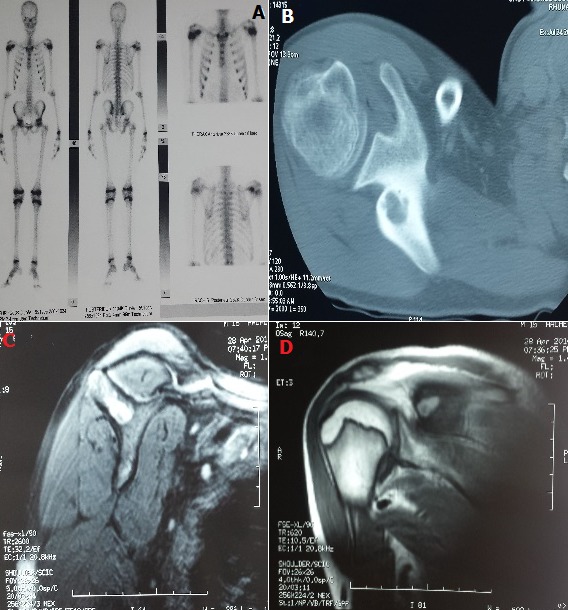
A) bone scintigraphy: hyperfixation of the radiotracer regarding the right scapular spine evoking an osteoid osteoma; B) CT scan of the right scapula: a very limited osteolytic lesion without osteocondensation edges with a non-enhanced hypodense matrix after injection of contrast agent; (C,D) MRI of the scapula: lesion of the right scapular spine in hypersignal T1 and T2 without cortical rupture or periosteal reaction suggestive of a benign lesion such as osteoid osteoma
